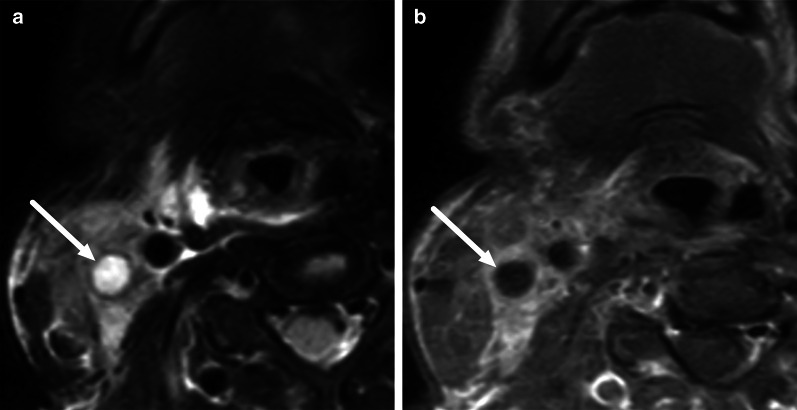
Thrombosis of the internal jugular vein (arrows) associated with Lemierre’s syndrome in a teenager with a throat infection, demonstrated on axial fat-suppressed T2-weighted (a) and post-contrast T1-weighted (b) images. Thrombosis may be difficult to detect on standard MRI sequences. Here, the lack of flow void is seen on the T2-weighted image (a), whereas the hypointense thrombus is difficult to separate from the flow void on the T1-weighted image (b)
